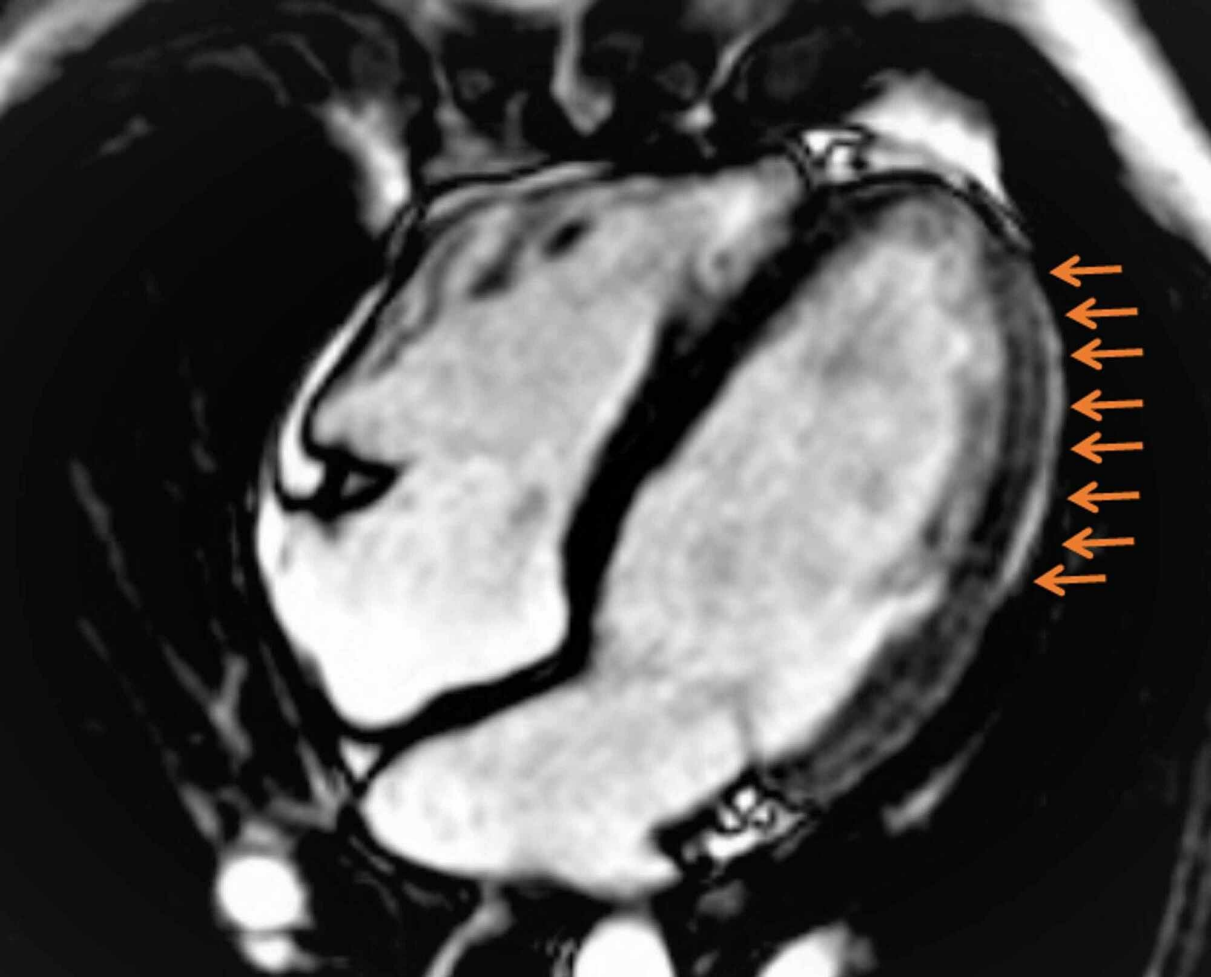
Cardiac MRI showing extensive sub-epicardial lateral wall LGE (shown by arrows) in keeping with myocarditis. MRI: magnetic resonance imaging, LGE: late gadolinium enhancement.
